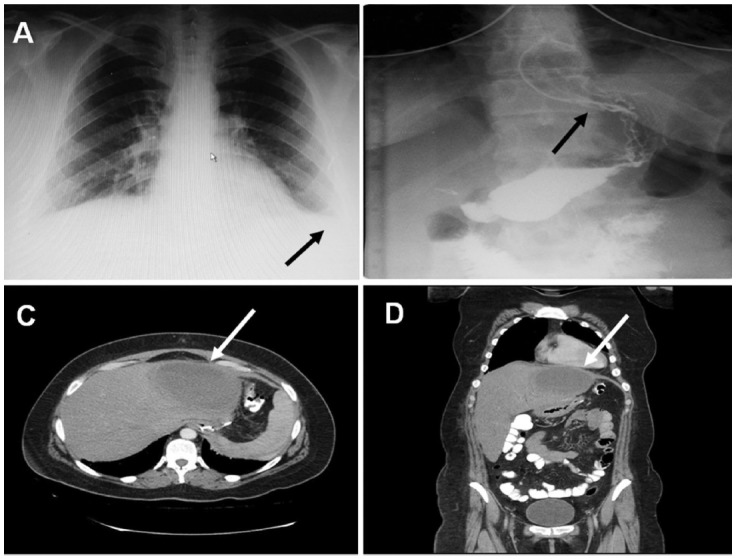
A) Chest X-ray showed blunting of the left costaphrenic angle with minimal left pleural effusion, B) oral contrast given gastro duodenography which ruled out staple line leak, C) Axial section of the liver abscess, D) Vertical image of the liver abscess.
